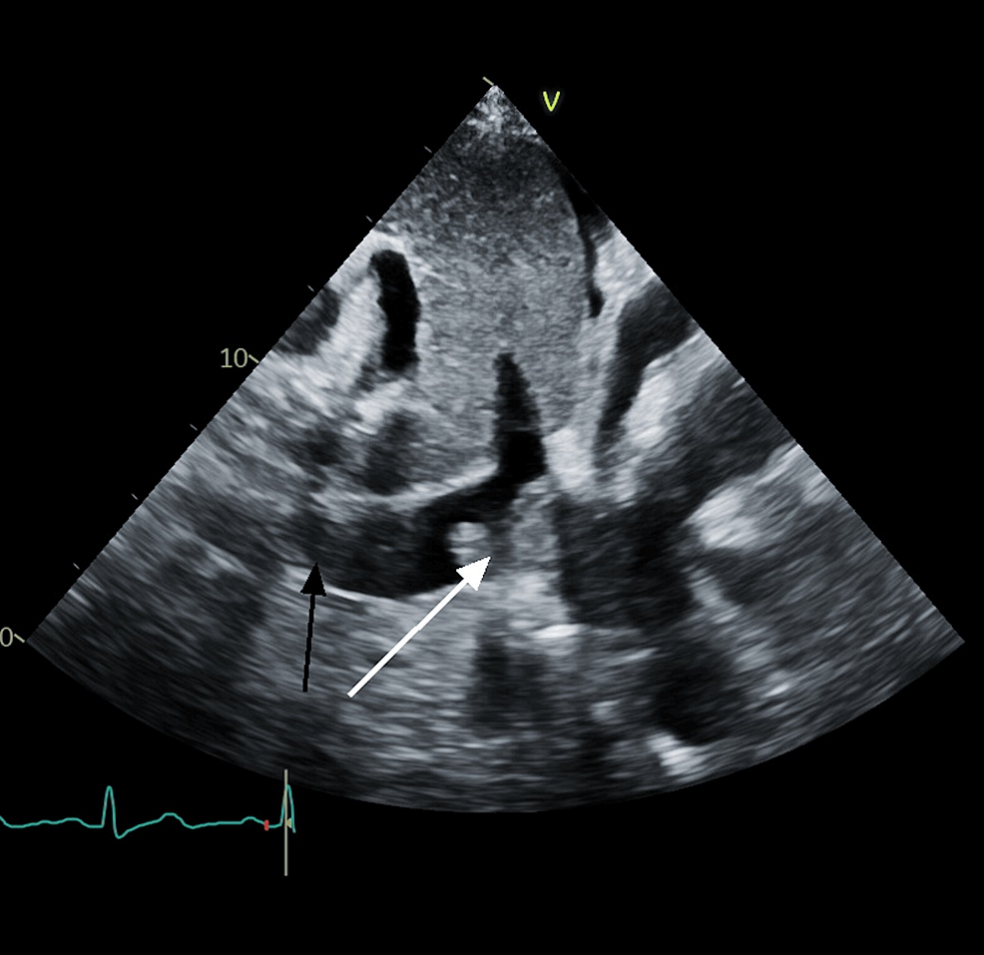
IVC thrombus on echocardiogram The black arrow indicates inferior vena cava (IVC), and the white arrow shows a large thrombus in IVC.
